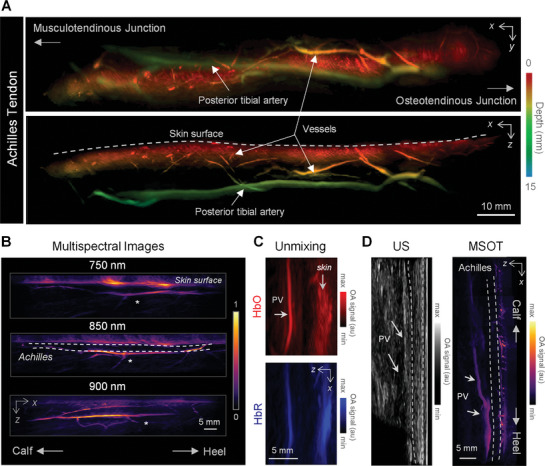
Characterization of the Achilles tendon. A) Volumetric MSOT image of the Achilles tendon shown projected in xy and xz planes. Depth was colour‐coded where red signifies the most superficial structures and blue the deeper structures. The posterior tibial artery and vessels surrounding the Achilles are labeled. B) MSOT scans of an Achilles tendon at 750, 850, and 900 nm wavelengths from the heel to the calf, where * depicts the same anatomical location. Deeper vessels become more visible for the longer wavelengths. C) Section of the Achilles tendon spectrally unmixed for bio‐distribution of oxygenated (HbO) and deoxygenated (HbR) hemoglobin. Both components are present in the PV. D) B‐mode ultrasound and MSOT images of the Achilles tendon of the same volunteer for anatomical validation. The Achilles tendon and the posterior vessel are both indicated.
